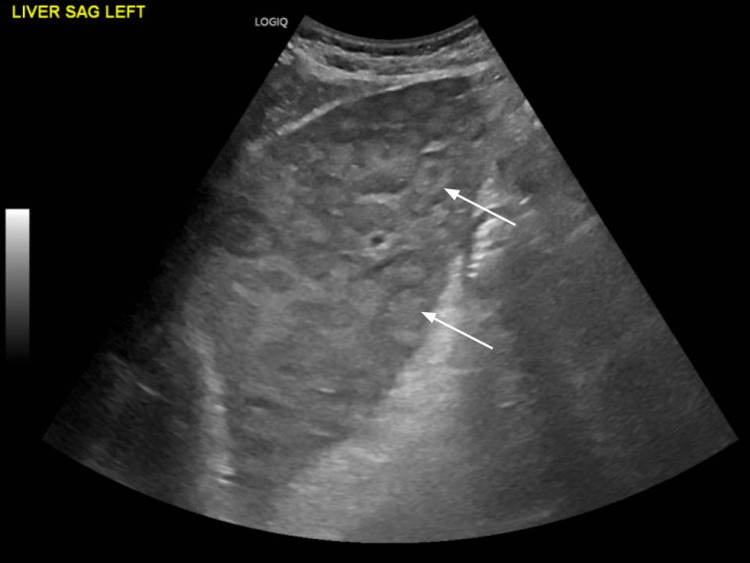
The ultrasound scan (US) of the abdomen revealed an enlarged liver, infiltrated with multiple hyperechoic rounded masses concerning the extensive metastatic disease
